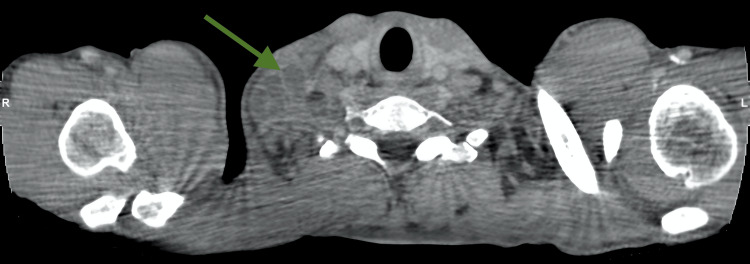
CT Neck and Trunk, axial view. Green arrow indicates enlarged right supraclavicular lymph node with necrotic centres
